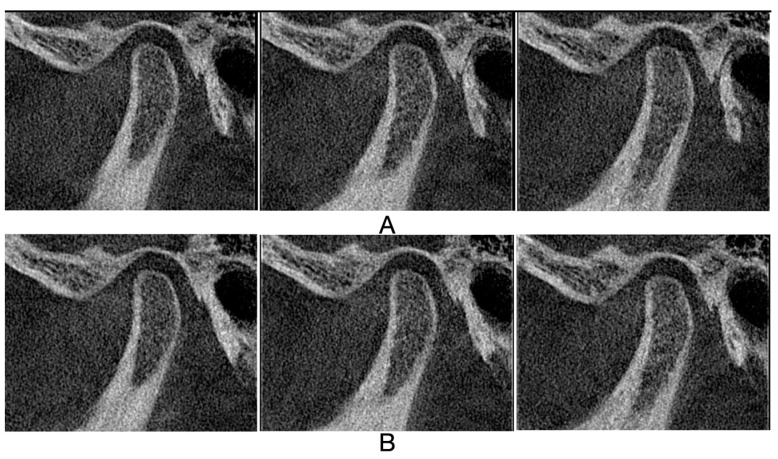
CBCT oblique sagittal view: (A) CBCT images before therapy: first, second, and third mid-sagittal slice of left condyle, no morphological changes: convex condylar shape, uninterrupted cortical bone, no cavity in the bone marrow; (B) CBCT images after therapy: first, second, and third mid-sagittal slice of left condyle, no morphological changes.
